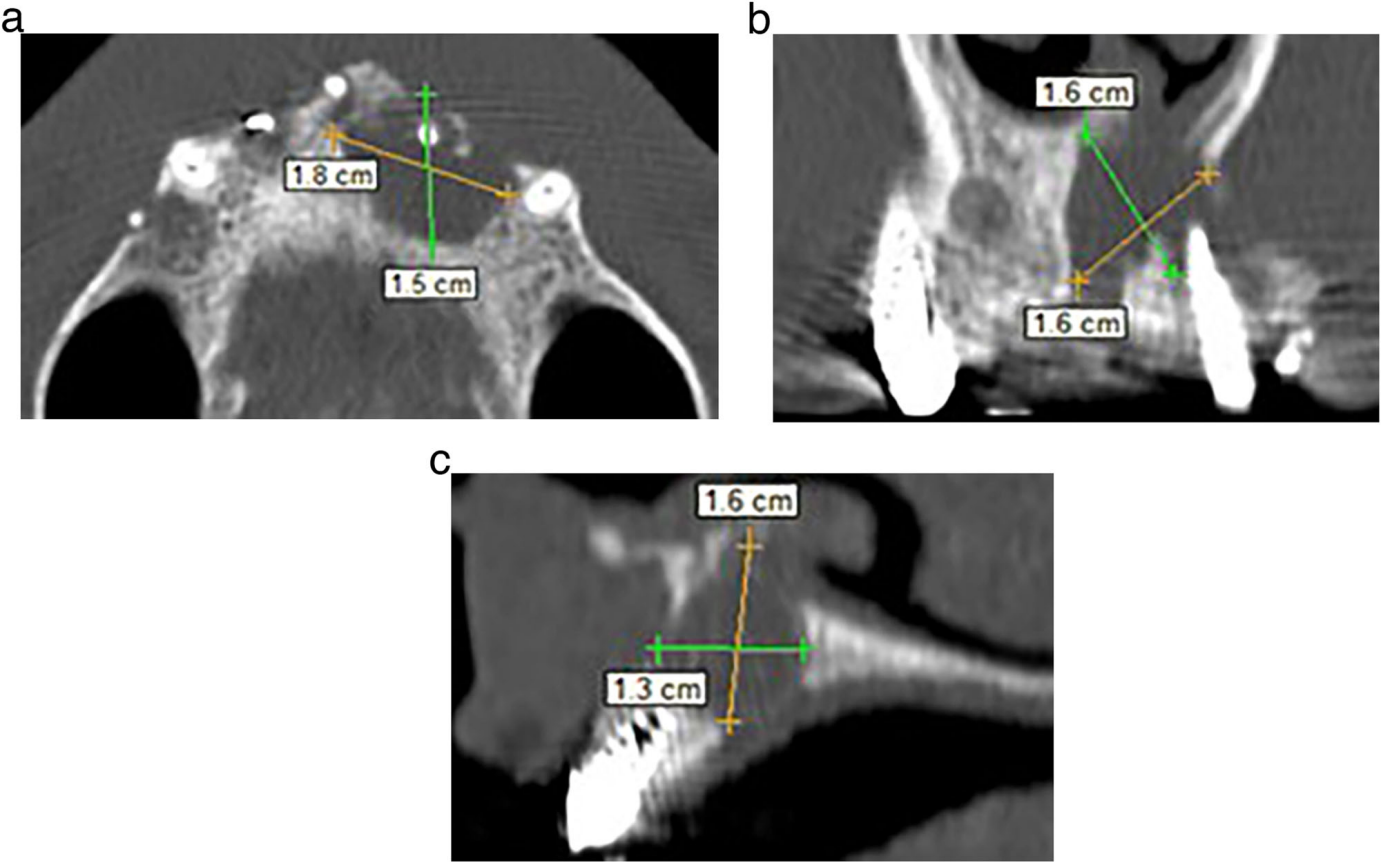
Outcomes measurement procedures. Determining the apical lesion diameter in CBCT image sections; a: in the Axial section, b: in the Coronal section, and c: in the Sagittal section.
